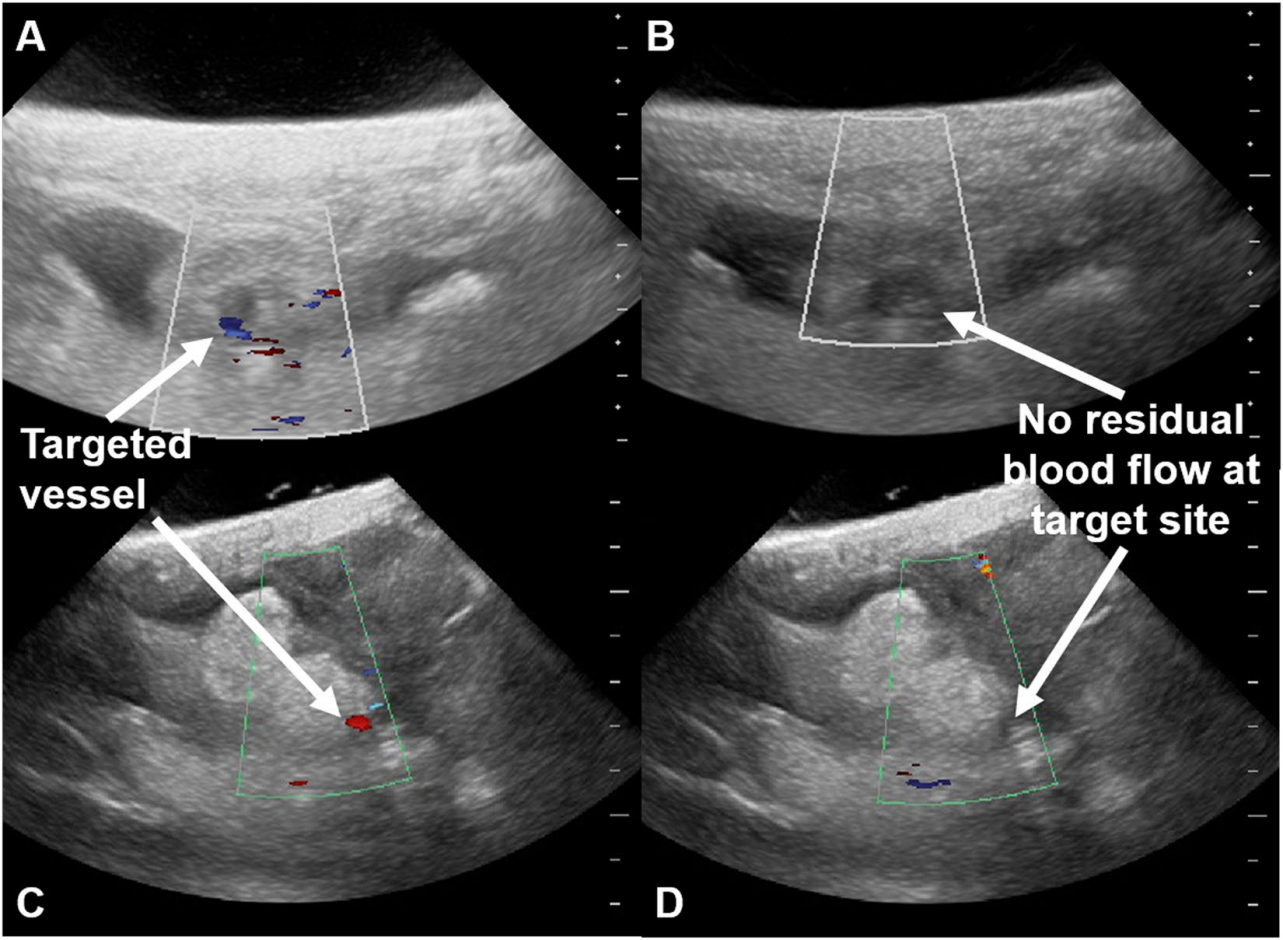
Colour flow Doppler imaging demonstrating successful placental vascular occlusion. (A,C) Pre-exposure colour flow Doppler imaging of a placentome. The intended vascular target is labelled. The colour flow Doppler gain is −25.0–25.0 cm.s^−1^ in image (A) and -13.0–13.0 cm.s^−1^ in image (C). Images were obtained using a 4–10 MHz phased sector array transducer (P10–4, Z.one Zonare ultrasound system, Mindray, China). (B) Post-HIFU exposure colour flow Doppler imaging of the placentome shown in image (A) demonstrating no colour flow Doppler signal “no flow” within the targeted vessel. The HIFU exposure series which resulted in occlusion comprised 6 transdermal exposures, 2 mm spaced, 5 s duration, 5 s interval, estimated *in situ* intensity 3.0 kW.cm^−2^. (D) Post- HIFU exposure colour flow Doppler imaging of the placentome shown in image (C) demonstrating “no flow” within the targeted vessel. The HIFU exposure series which resulted in occlusion comprised 6 transdermal exposures, 2 mm spaced, 5 s duration, 5 s interval, estimated *in situ* intensity 4.1 kW.cm^−2^.
